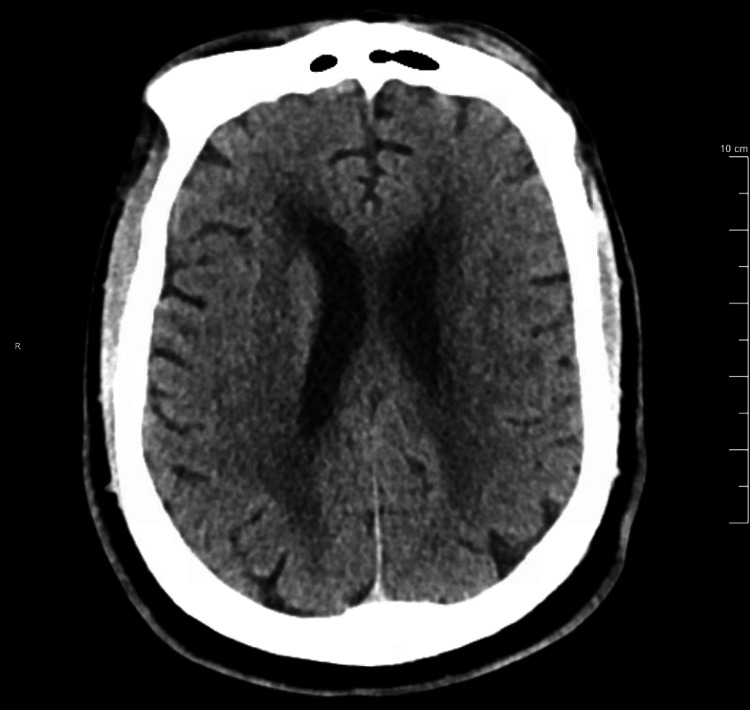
Computed tomography (CT) scan without contrast of head showing no acute process
